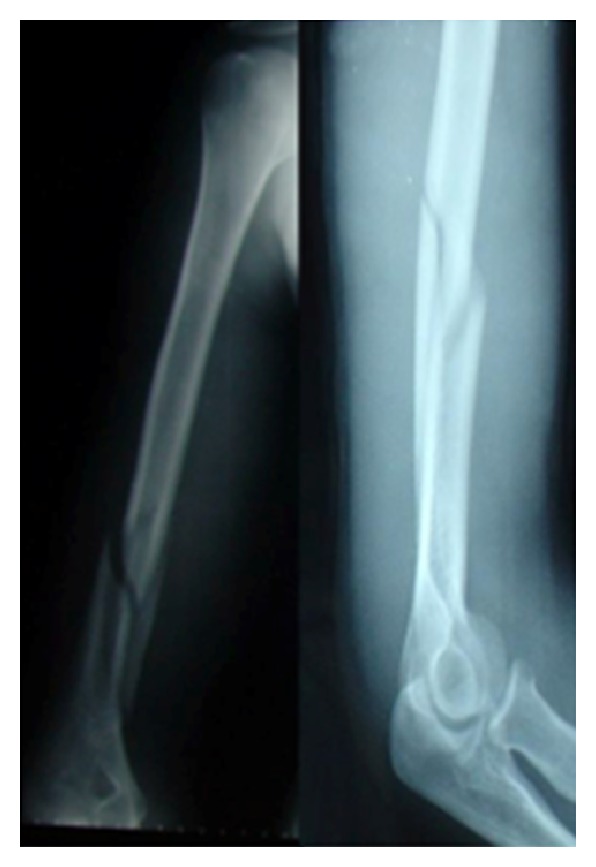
X-rays at the first application to the emergency service.
